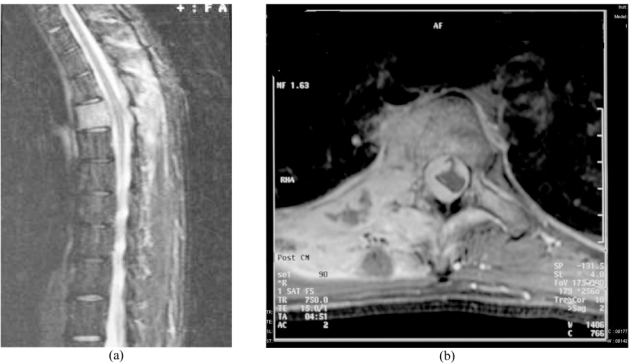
a) Sagittal MRI (STIR, TR 3586.0 TE 60.0) showed abnormally increased signal intensity in the T4 vertebral body and the subcutaneous tissues of the thoracic paravertebral region; b) Axial Post gadolinium image revealed heterogenous enhancement of the vertebral body, right pedicle, laminae, and paravertebral soft tissues at the T4 vertebral level.
